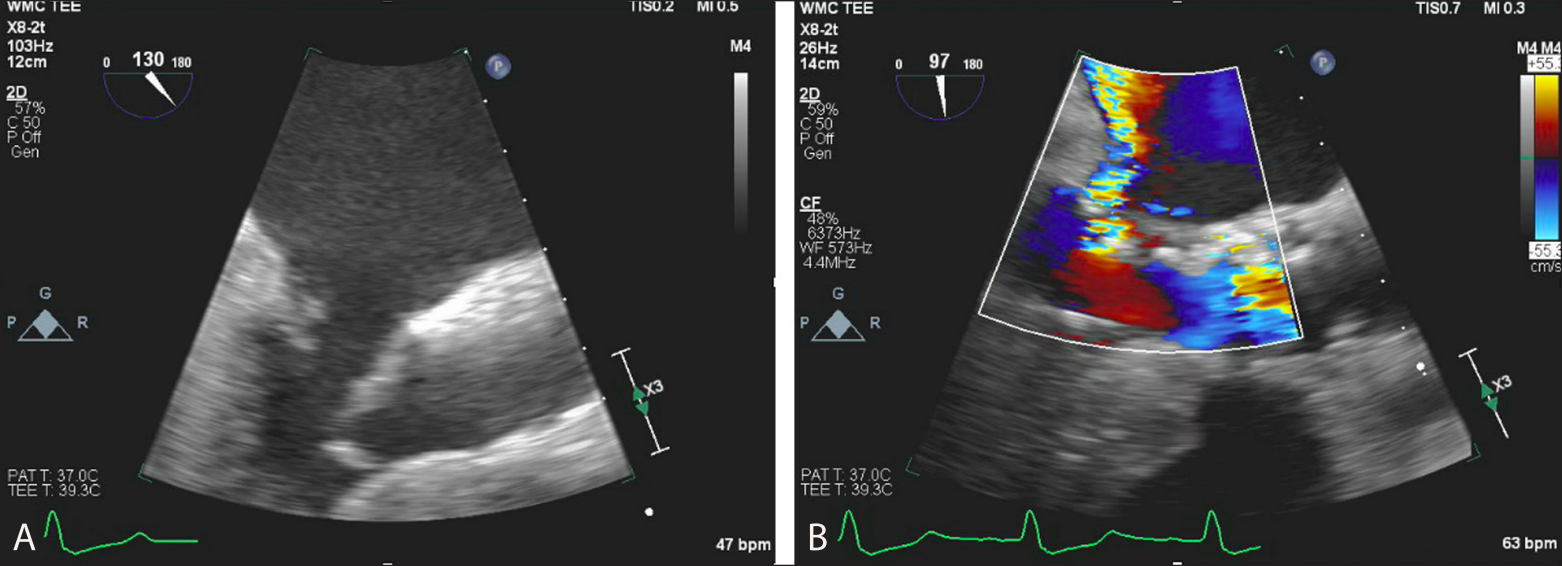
Transesophageal echocardiography showing (A) chordal rupture and (B) severe mitral regurgitation.
